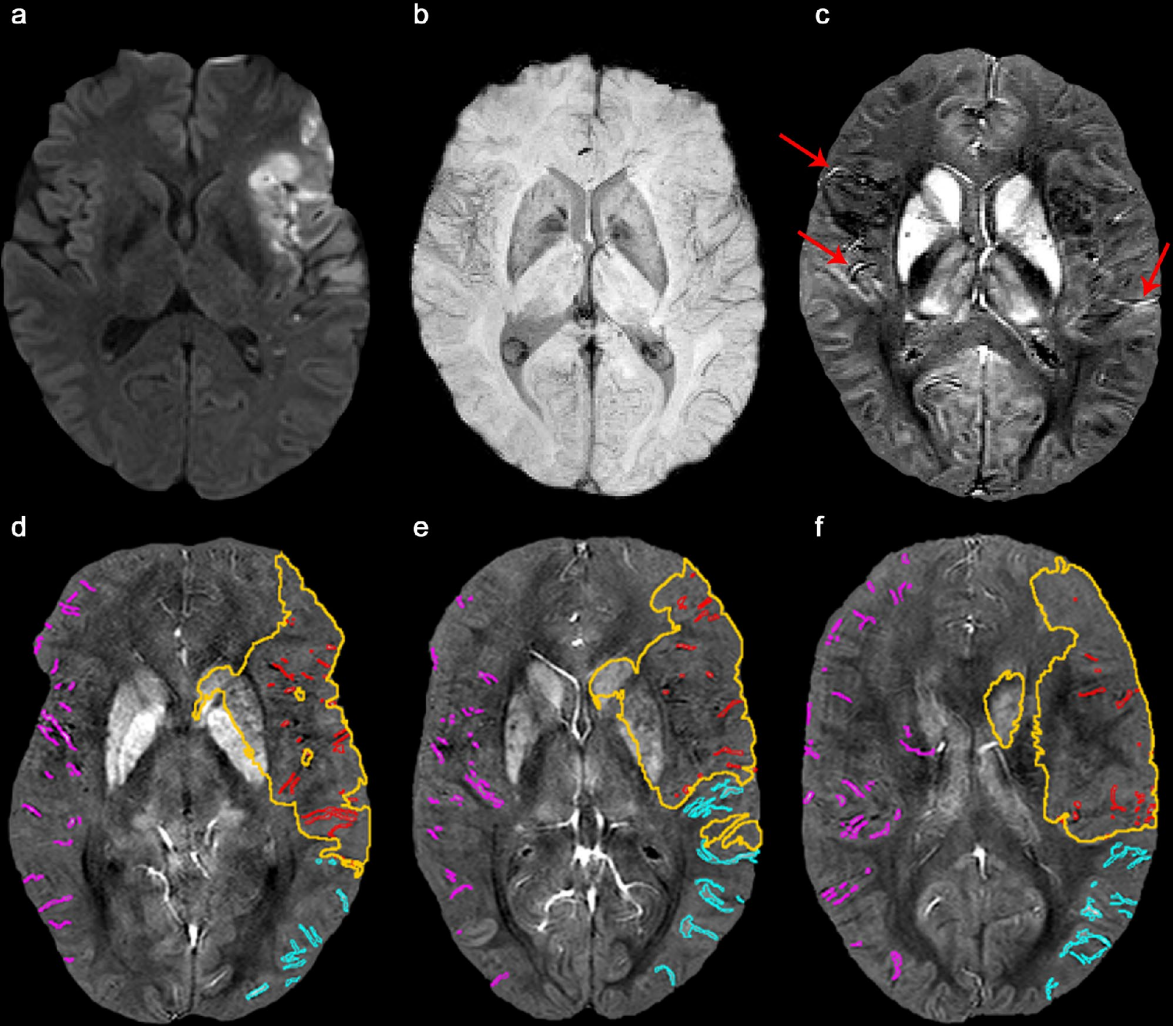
MR images of a stroke patient aged 49 years with successfully recanalized MCA-M1 occlusion on the left hemisphere. The DWI (a) shows the hyperintense infarct area on the left hemisphere. The SWI (b) helps identifying the localization of vessels. The corresponding susceptibility map is depicted in (c). Veins with higher levels of paramagnetic deoxyhemoglobin content appear brighter (red arrows). Representative susceptibility images covering the MCA territory are shown in (d–f). Cortical veins are delineated in different colors depending on the territory. Within the stroke area (yellow line) defined by DWI cortical veins are delineated in red, those within the ipsilateral non-ischemic MCA territory in cyan and those in the contralateral MCA territory in magenta.
